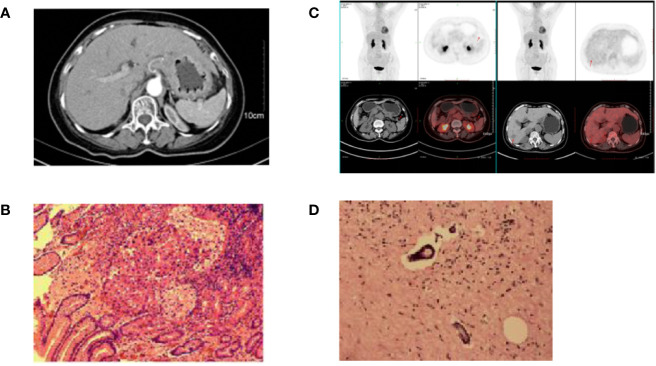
Pathology and imaging data of patient 2. (A) The CT of the patient at the first examination; (B) The gastroscopic pathology that suggested gastric poorly differentiated adenocarcinoma with abdominal lymph node metastasis; (C) PET-CT after four cycles of combined chemotherapy showed no focal increase in FDG in local gastric wall of gastric cardia and fundus; (D) Postoperative pathology of the patient.
